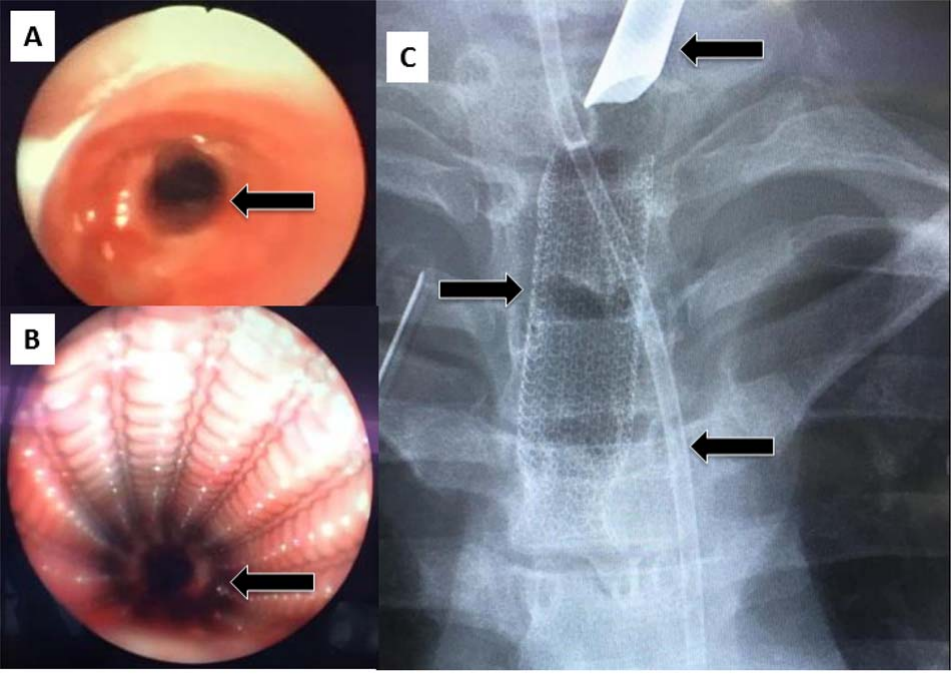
(A) Rigid bronchoscopy show 4 mm stenosis of the distal trachea, (B) intra-tracheal showing the stent following dilatation and placement of stent, (C) plain X-ray showing stent in place, feeding tube and endotracheal tube.
